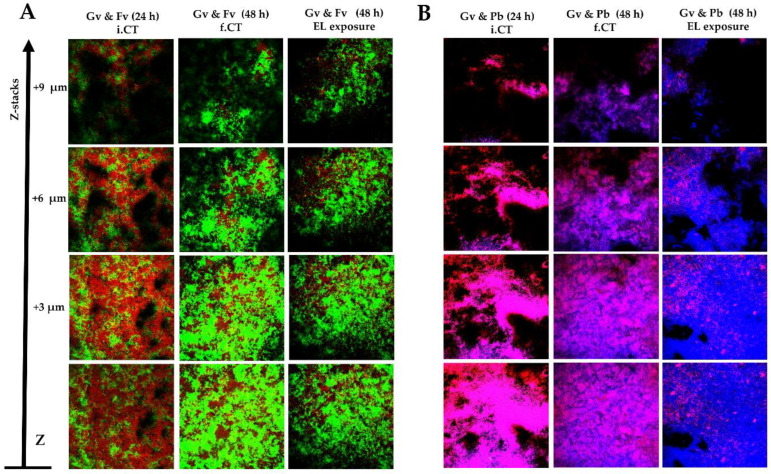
Representative CLSM image showing the organization of the dual-species BV-associated biofilms. (A) An example data set on the organization of Gv and Fv dual-species biofilms. (B) An example data set on the organization of Gv and Pb dual-species biofilms. Gv and Fv cells were differentiated by hybridization with PNA Gard162 (red/purple color when coupled with DAPI) and AtoITM1 probes (green color), respectively, while Pb and non-hybridized Gv cells were differentiated by DAPI (blue color). Abbreviations: *Gardnerella vaginalis* strain ATCC 14018^T^ (Gv); *Fannyhessea vaginae* strain ATTC BAA-55^T^ (Fv); *Prevotella bivia* strain ATCC 29303^T^ (Pb); endolysin (EL); initial control at pH7 before the medium replacement (i.CT); final control at pH5 after incubation with fresh medium (f.CT).
